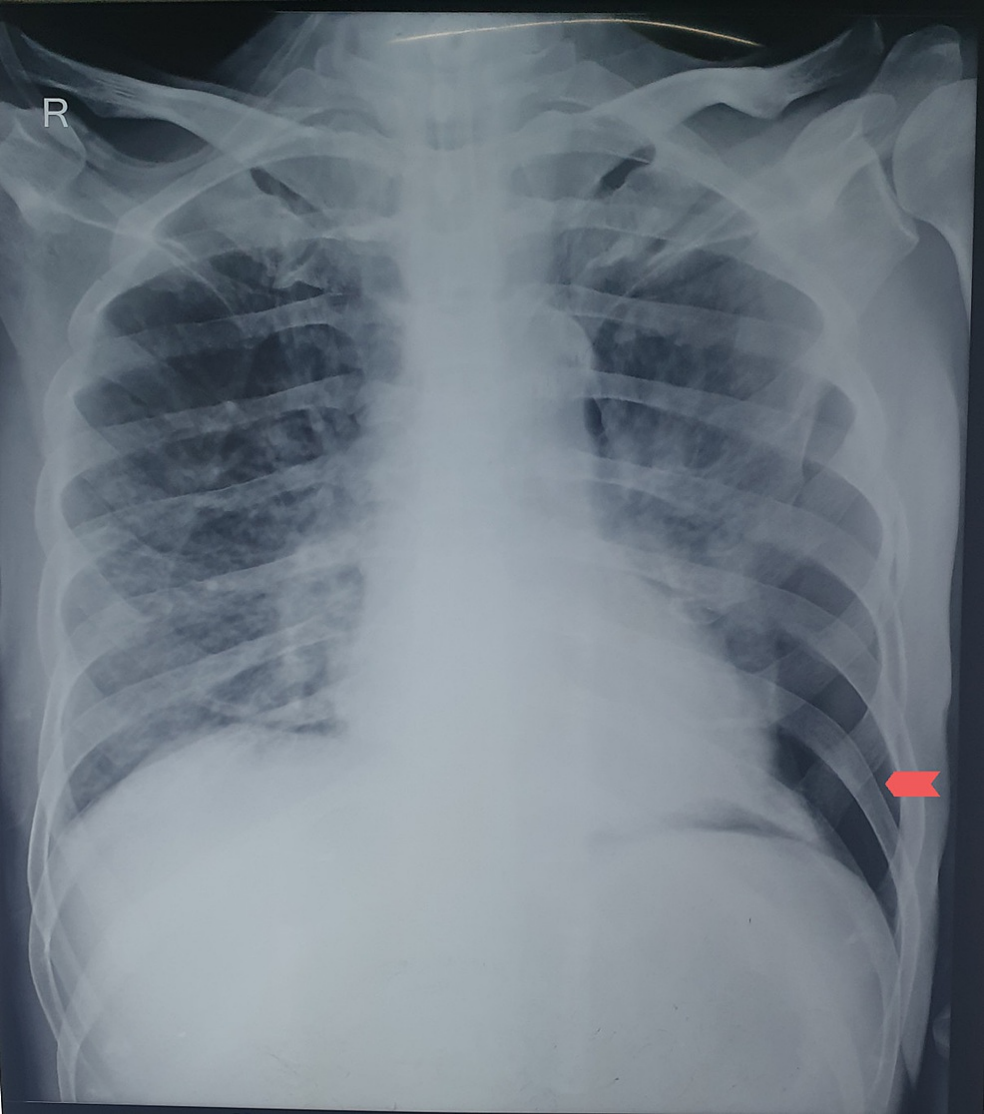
CXR demonstrating left-sided pneumothorax (shown by arrow). CXR, chest X-ray
